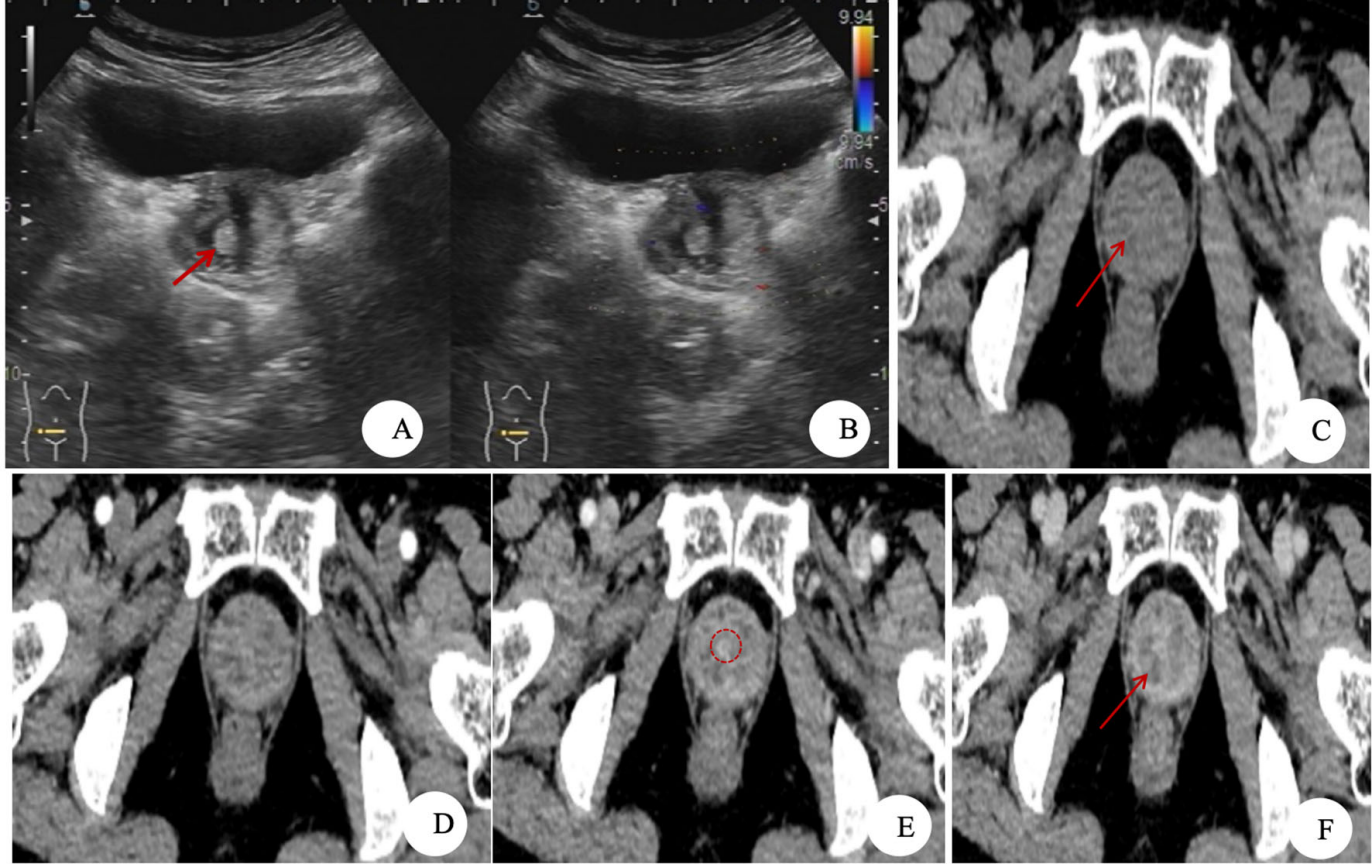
Images of clear cell adenocarcinoma of the urethra (CCAU). (A,B) Ultrasonographic images. (A) An irregular mass with ill-defined margins is observed in the post-bladder urethral region, demonstrating heterogeneous echogenicity. A small hyperechoic area (red arrow) and an anechoic zone are visible within the mass. (B) Color Doppler flow imaging (CDFI) demonstrates scant blood flow signals within the mass. (C–F) CT images. (C) Non-contrast CT: A soft tissue density mass is observed in the posteroinferior urethral region to the bladder, with partially ill-defined margins. Patchy areas of low density (red arrow) are noted within the mass. (D–F) Contrast-enhanced CT, arterial, venous, and delayed phases: The mass demonstrates marked heterogeneous enhancement. Patchy non-enhancing necrotic areas (red arrow) and a circumferentially enhancing urethral image (red dashed circle) are visible within the mass.
